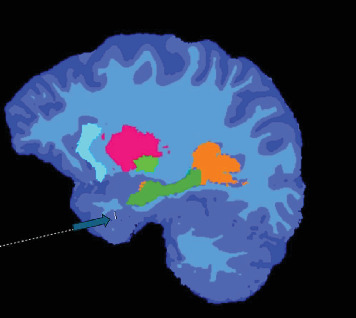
Coronal T1-weighted MR-images depicts a segmentation of the piriform cortex.
